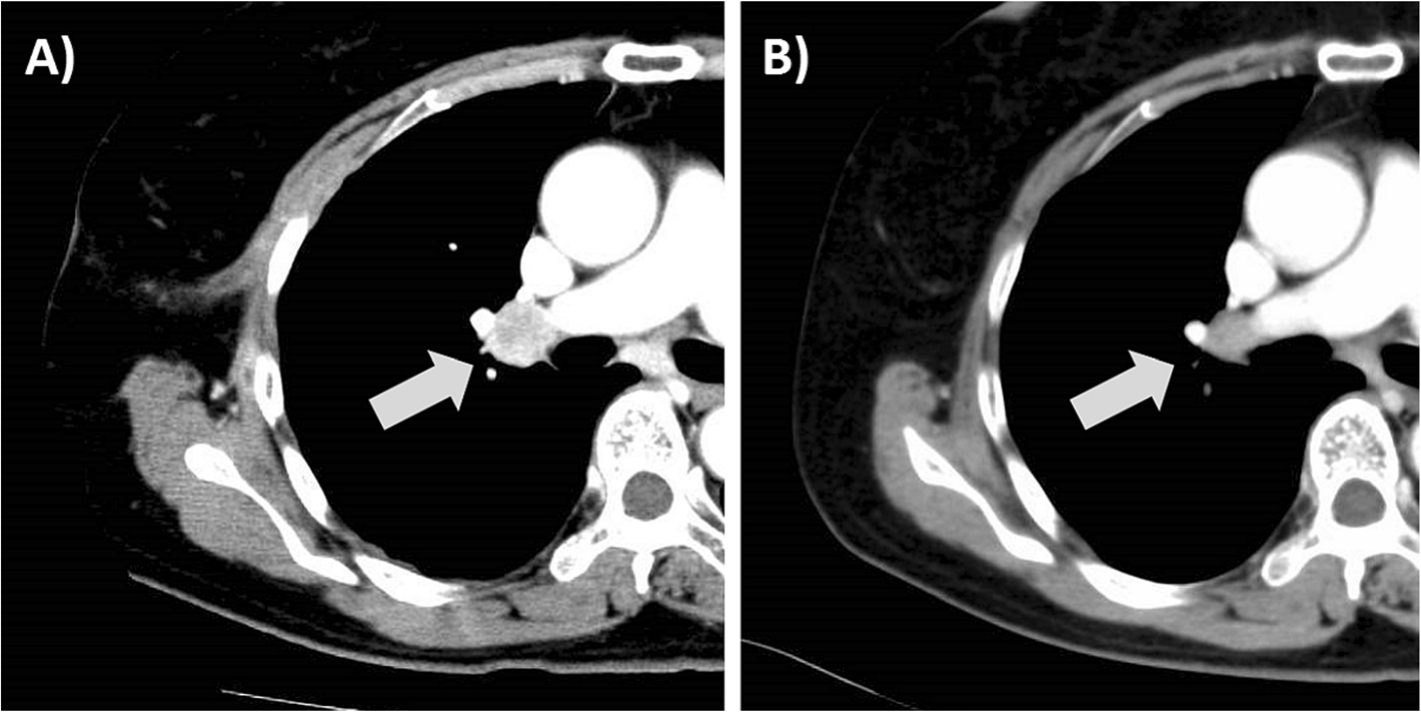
Hilar lymph node metastases appeared 6 years after initial treatment of the primary tumor. A) A contrast-enhanced computed tomography image of the patient’s chest performed in July 2003. The arrow indicates a contrast-enhancing enlarged lymph node. Serum tumor markers are elevated as follows: carcinoembryonic antigen was 43.8ng/mL, carbohydrate antigen 19–9 was 151U/mL, and carbohydrate antigen 125 was 343U/mL in June 2003. B) The lymph node (arrow) decreased to 65% of its peak volume in June 2005, 2 years after the recurrence. Serum tumor markers were decreased as follows: carcinoembryonic antigen was 27.1ng/mL, carbohydrate antigen 19–9 was 27U/mL, and carbohydrate antigen 125 was 188U/mL.
